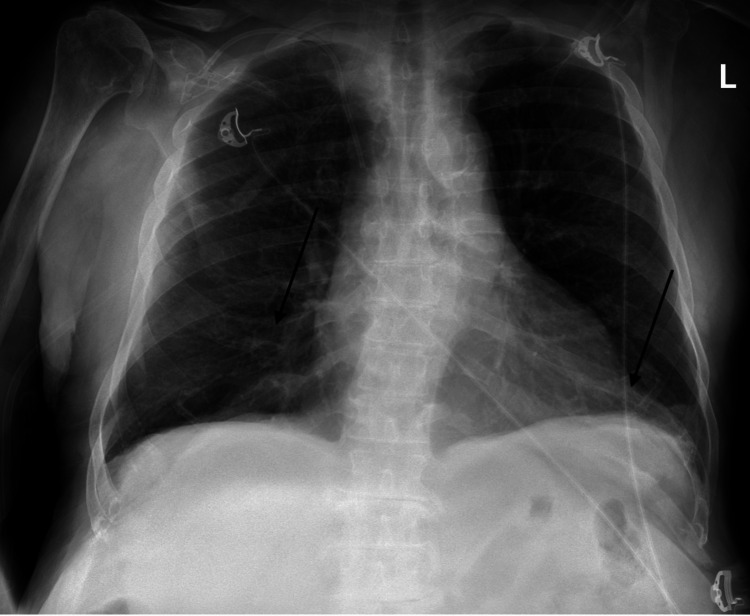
Chest X-ray on admission The arrows indicate bilateral pulmonary edema. There is no evidence of substantial pleural effusions.
